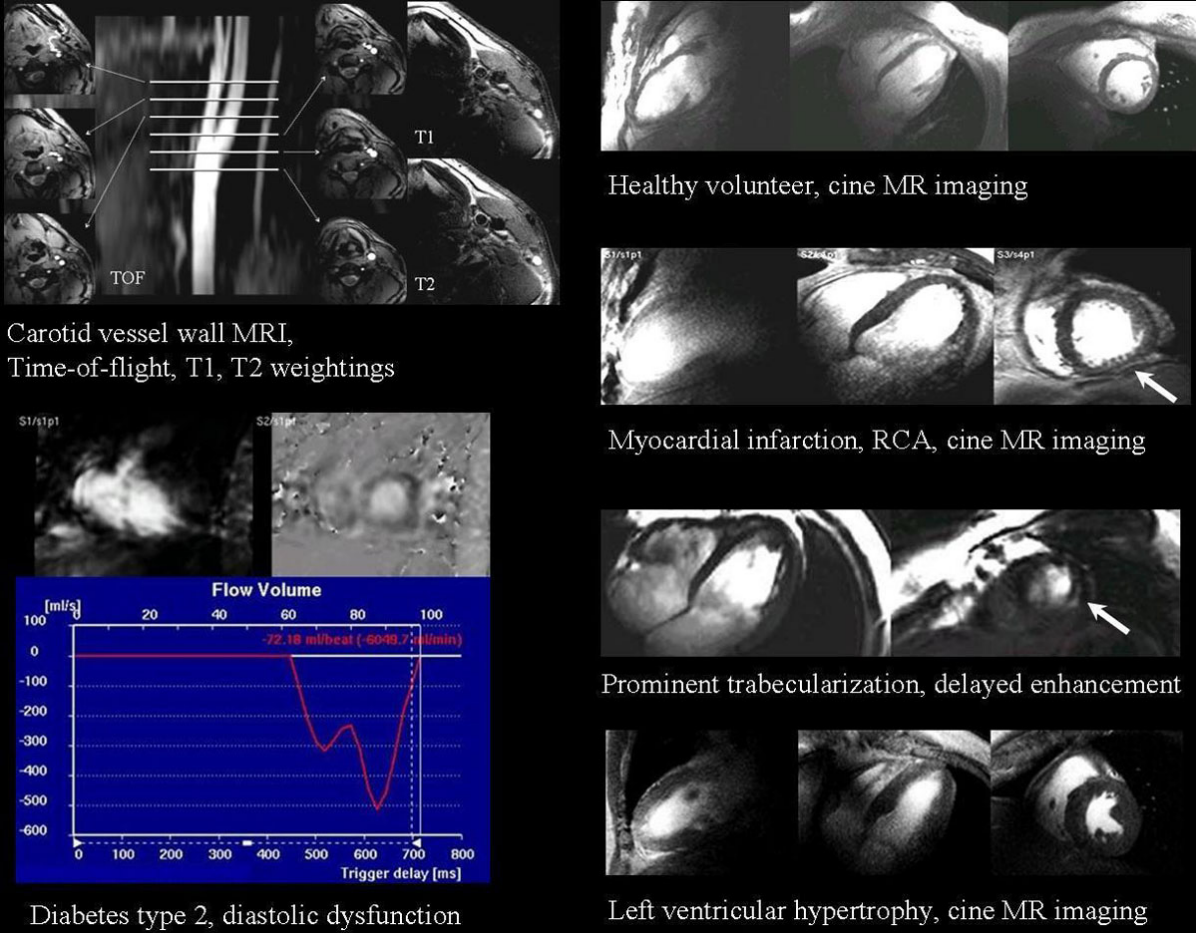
Examples of initial clinical experience with 7T Cardiovascular MR imaging. See text below images for clinical explanation, see abstract text for technical details.
